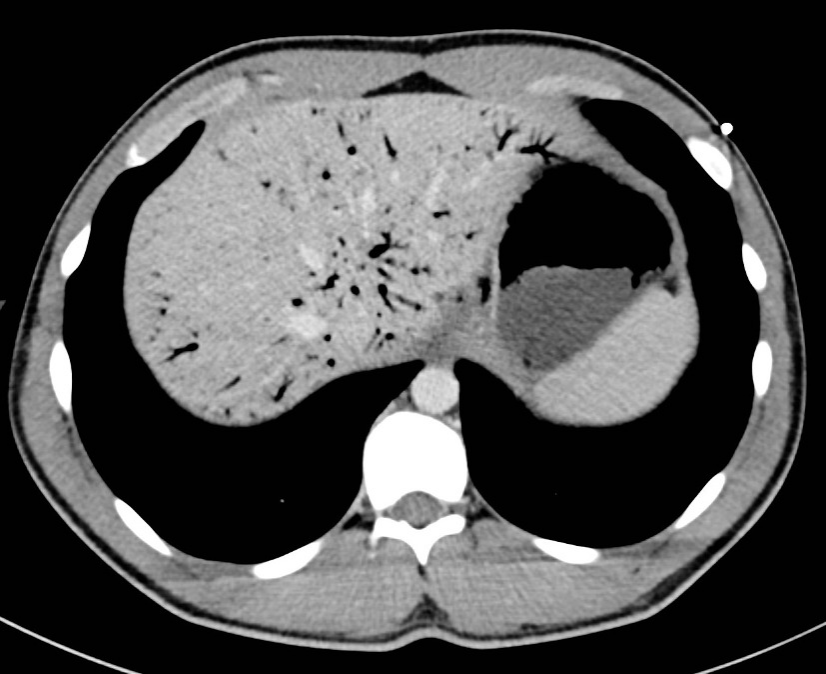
Axial portal venous phase CT image showing gastric mucosal thickening with portal venous gas.
